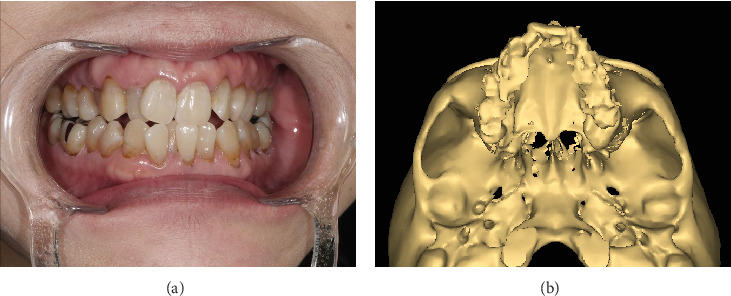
Preoperative photograph and three-dimensional computed tomography results (a and b), showing stenosis of the maxillary dental arch.
